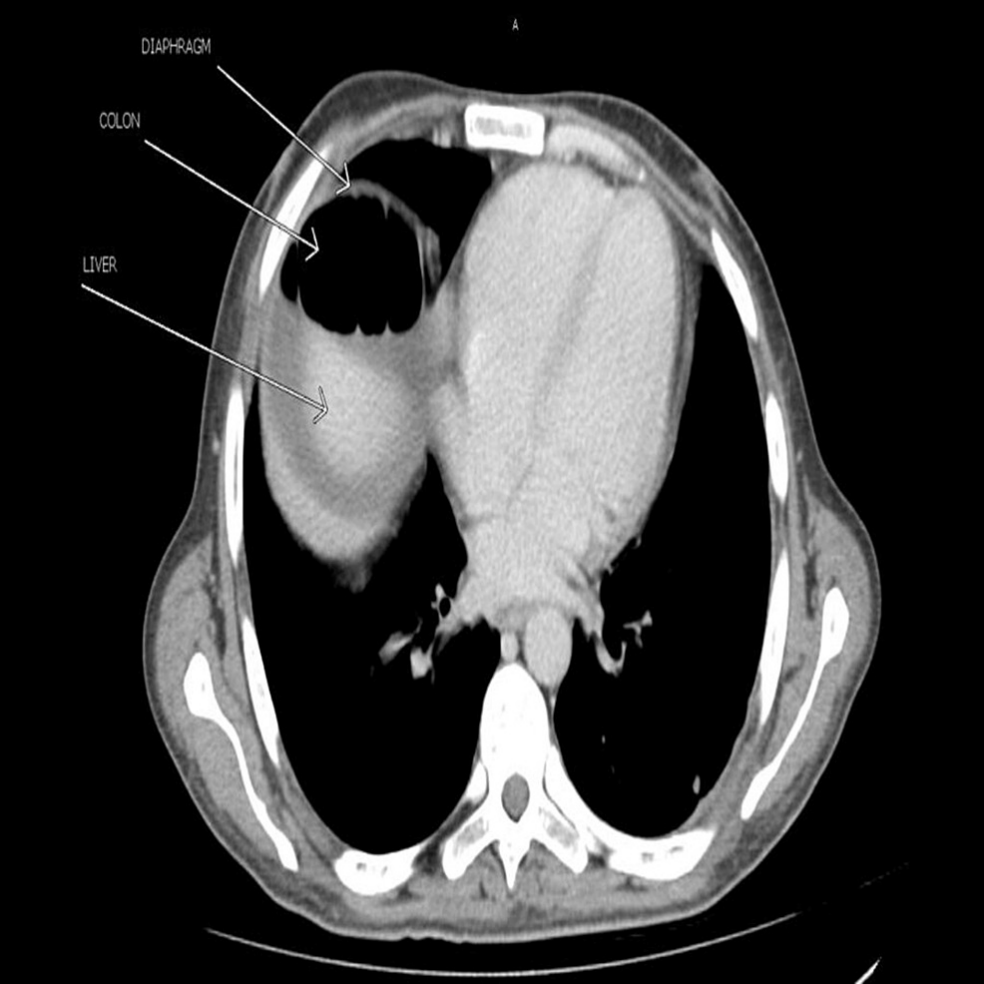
CT scan showing the interposition of the colon between the liver and diaphragm
